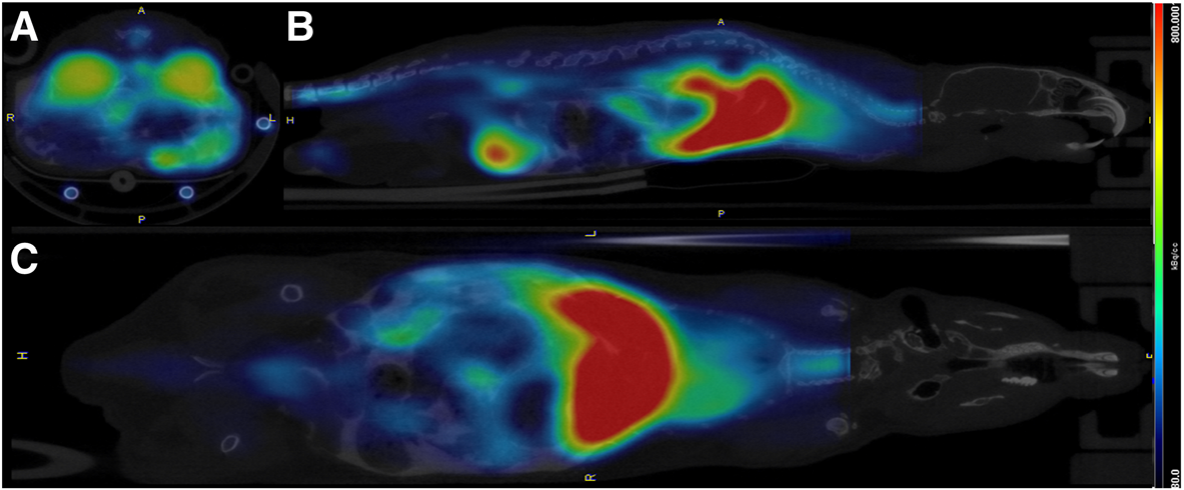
Transverse (A), sagittal (B), and coronal (C) planes of merged average PET/CT image of a representative subject. PET image was averaged across all frames and smoothed using a Gaussian kernel of 1 × 1 × 2 mm in PMOD. The color scale was set to the range 80 to 800 kBq/cc in all planes (Brain outside FOV).
